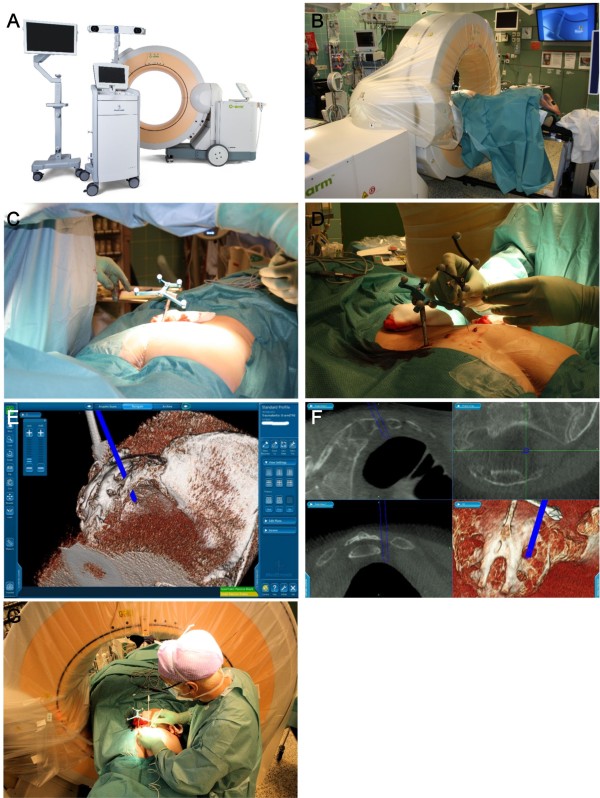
Intraoperative figures of sacral nerve stimulator lead implantation. The O-arm is a transportable CT-like scanner. With automated positioning, it can be easily placed around the patient on the radiolucent table to obtain 2D fluoroscopic images and 3D scanning in desired directions, and repeated when necessary (A). The patient’s position can be adjusted optimally to achieve a surgically and anesthetically comfortable situation (B). Patient tracker fixed to the bone (C). The multipurpose SureTrack instrument tracker was fixed to the needle and used for navigation (D). Needle inside S3, 3D image (E). The sacral structures are clearly visible in the O-arm images; it was possible to guide the instruments used in the correct direction and to the right depths. Inserts with 2D and a 3D images (F). Lead implantation; the O-arm has been moved in the cranial direction allowing free access to the operation area (G)*.*
